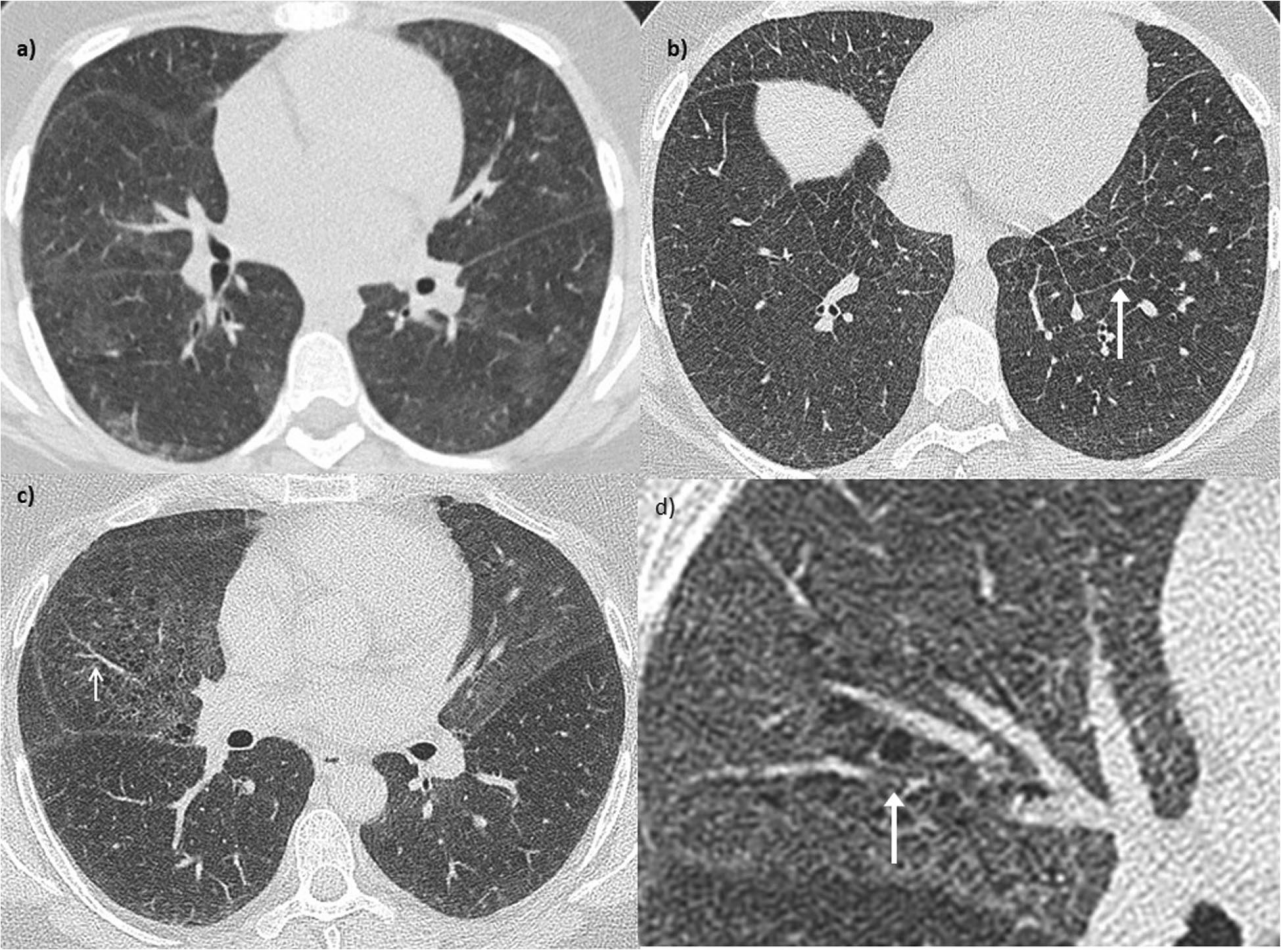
Patient 1. Radiologic evolution. Axial CT images of the lungs a) At 14 years old (the time of presentation), demonstrating a widespread ground glass infiltrate which has a geographic configuration; consisting of sharp demarcation between the infiltrate and normal lung. No interlobular septal thickening is evident. b) At 21 years old, the ground glass infiltrate appears more diffuse, and smooth interlobular septal thickening is present (arrow). c) At 31 years old, new emphysema is evident, in addition to a persisting ground glass infiltrate. d) Magnification view of C; the emphysema is seen to track along pulmonary vessels (arrow), indicating interstitial emphysema
